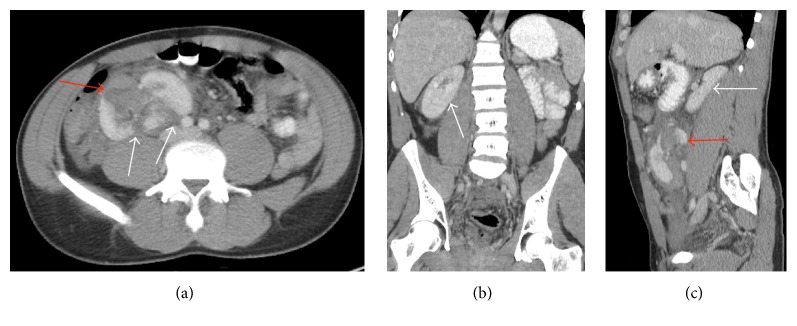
31-year-old male with crossed unfused renal ectopia. Axial contrast-enhanced CT image (a) shows lacerations (white arrows) through the posterior cortex of the ectopic left kidney, positioned anteriorly and inferiorly in the right abdomen. A perinephric hematoma (red arrow) is noted anteriorly. A coronal CT image (b) shows the uninjured right kidney in a normal location. A sagittal CT image (c) through the right abdomen shows the right kidney (white arrow) in a normal location in the renal fossa and the ectopic kidney (red arrow) in the lower right abdomen. Note the gap between these kidneys in this unfused ectopia.
